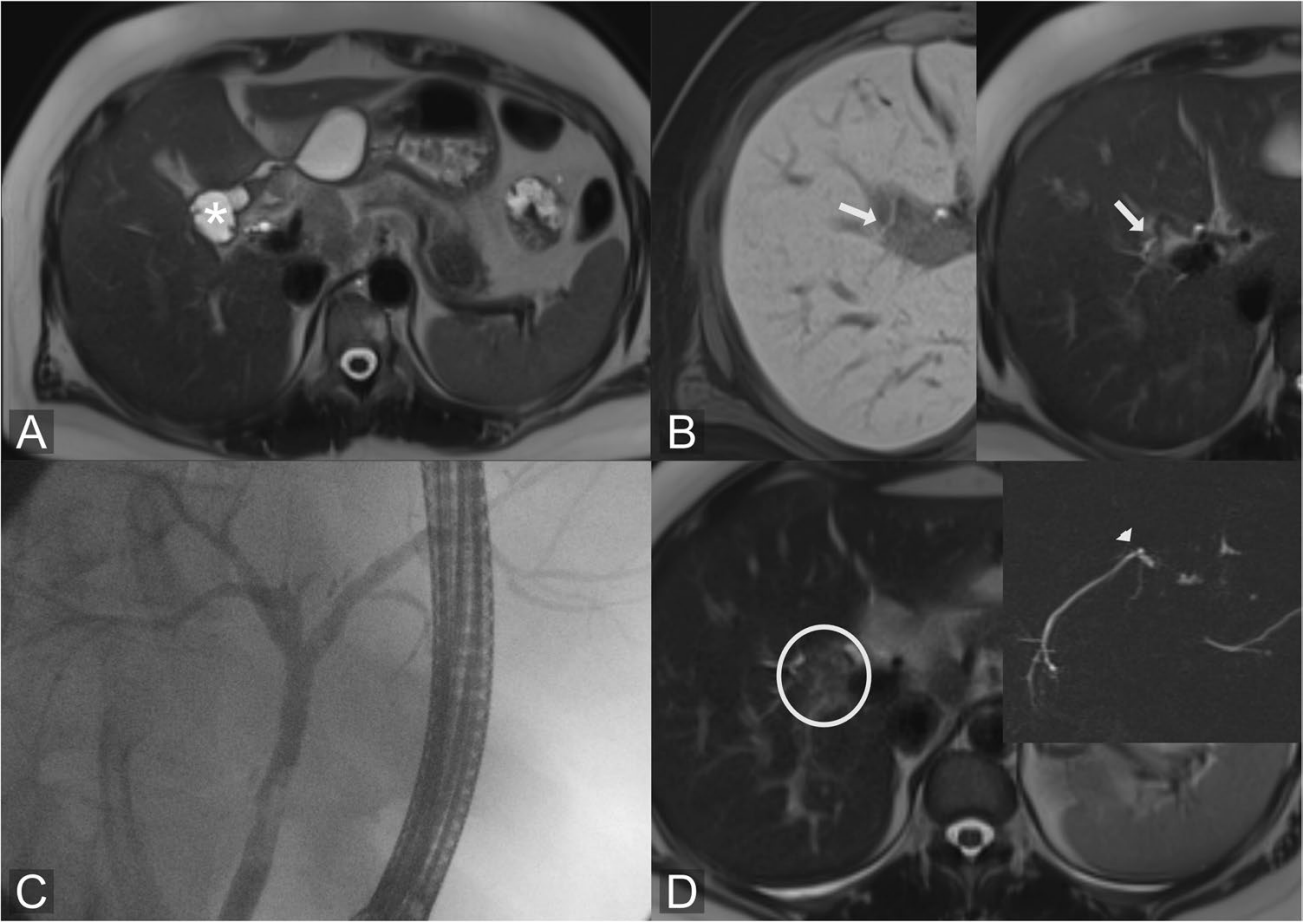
Aberrant duct: MRI of a 39-year-old patient after cholecystectomy. T2w image shows a biloma (asterisk) in the gall bladder fossa (A). Gd-EOB-DTPA-MRCP reveals contrast extravasation into the gallbladder fossa originating from an aberrant duct in liver segment 5 (arrow) which is also visible in T2w image (B). ERCP shows normal findings (C). Patient was treated by percutaneous biloma drainage. MRI 5 weeks after therapy shows complete regression of the biloma and post-inflammatory scar tissue at the former leakage site (circle), resulting in non-symptomatic dilatation of the corresponding aberrant bile duct in MRCP (arrowhead) (D)
